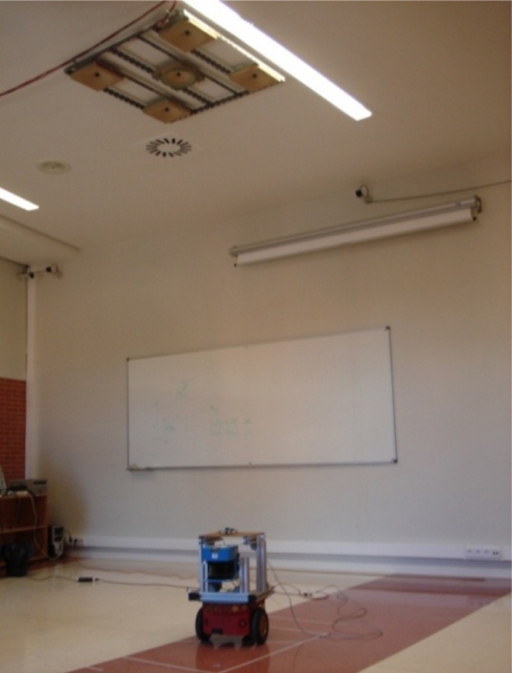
Ultrasonic LPS by GEINTRA used in experimental tests.
